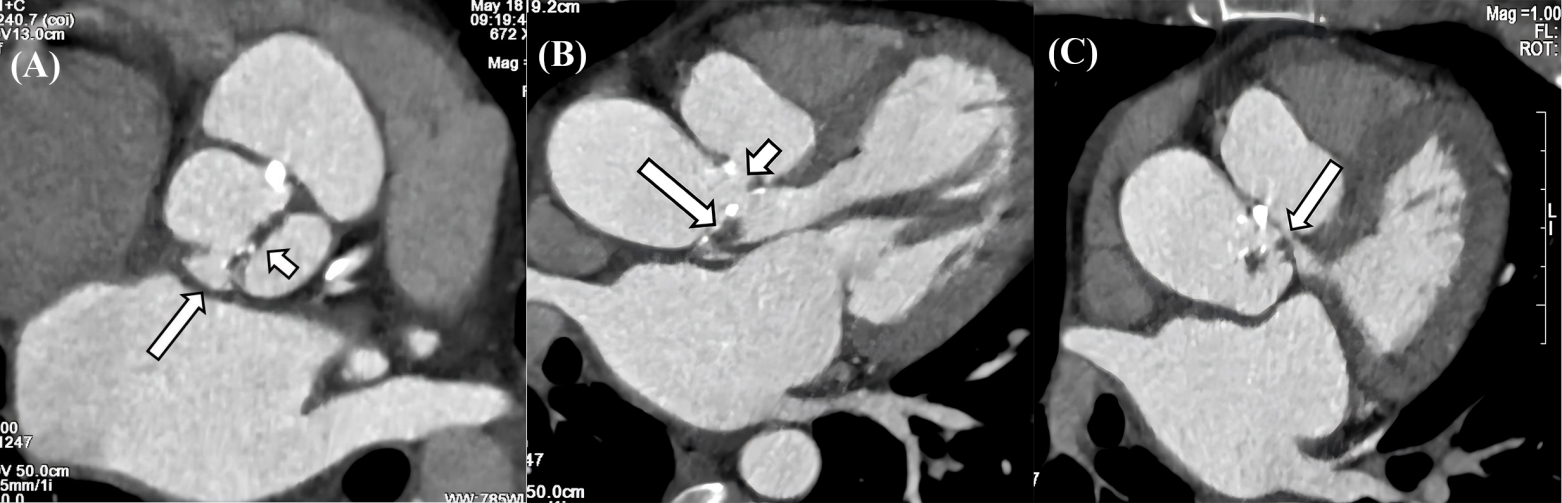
CT characteristics of IE. (A) Valve thickening, calcification
